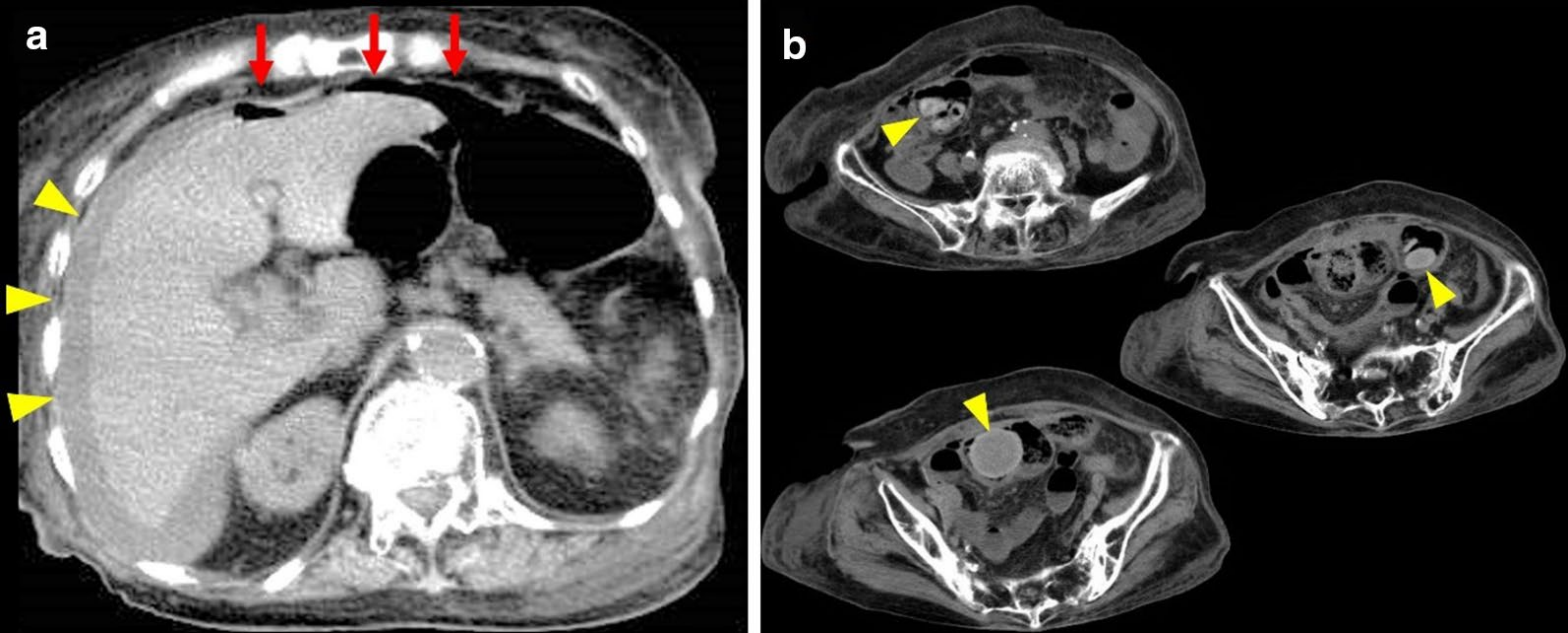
Abdominal plain computed tomography (CT) imaging performed at the first visit to a previous hospital. a Free air in the upper abdomen (red arrows) and fluid collection in the right subphrenic space (yellow arrowheads); b slightly high-density structures located in an uncertain part of the intestine (yellow arrowheads)
